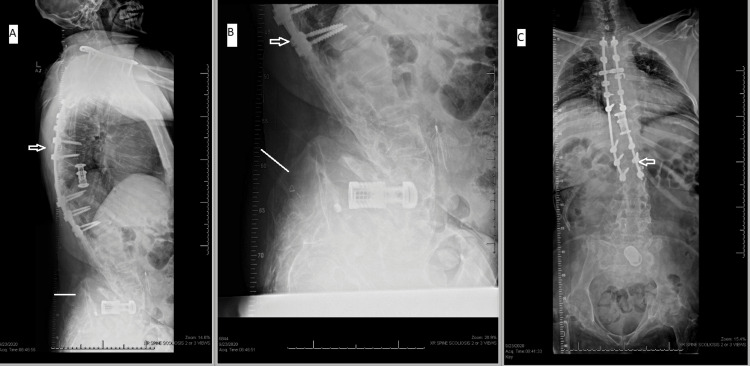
Preoperative radiographs and prior instrumentation A. Lateral view of the entire spine including previously installed hardware (arrow) and the superior region of the pelvis (line). B. Lateral view of the lumbar spine with instrumentation (arrow) and the pelvis (line). C. Anteroposterior radiograph of the entire spine and pelvis demonstrating prior instrumentation (arrow).
